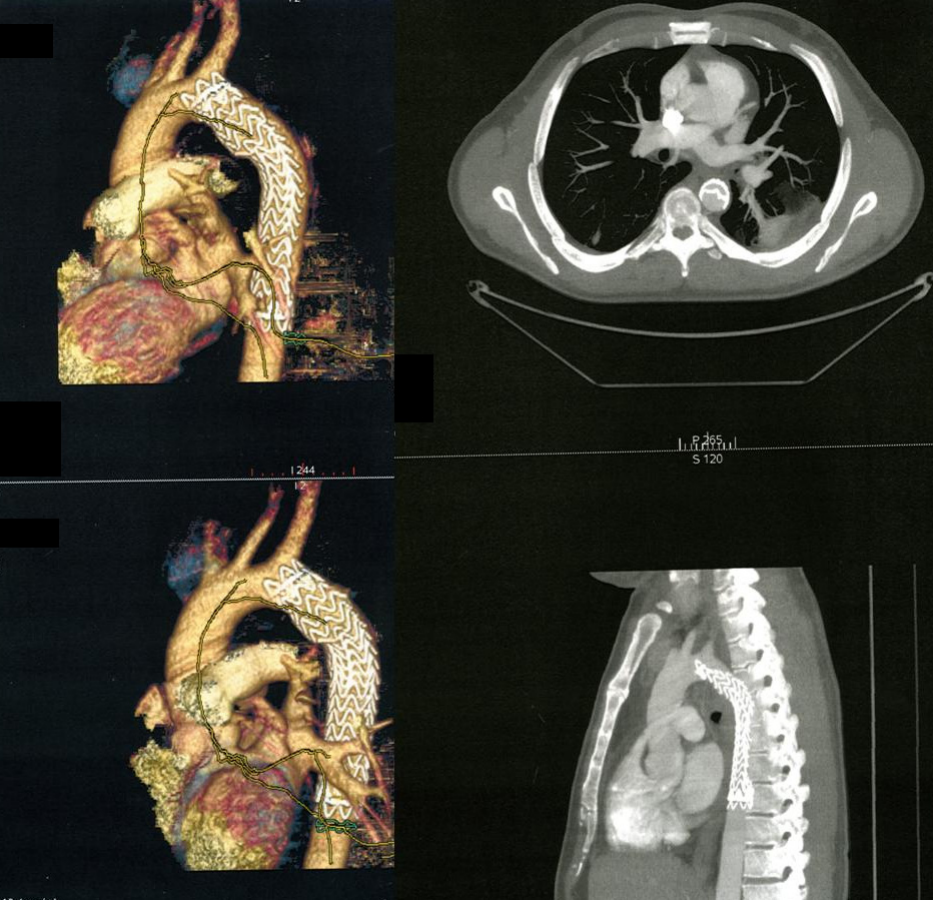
CT scan before discharge. Partial collapse of the endoprosthesis at the descending tract.
